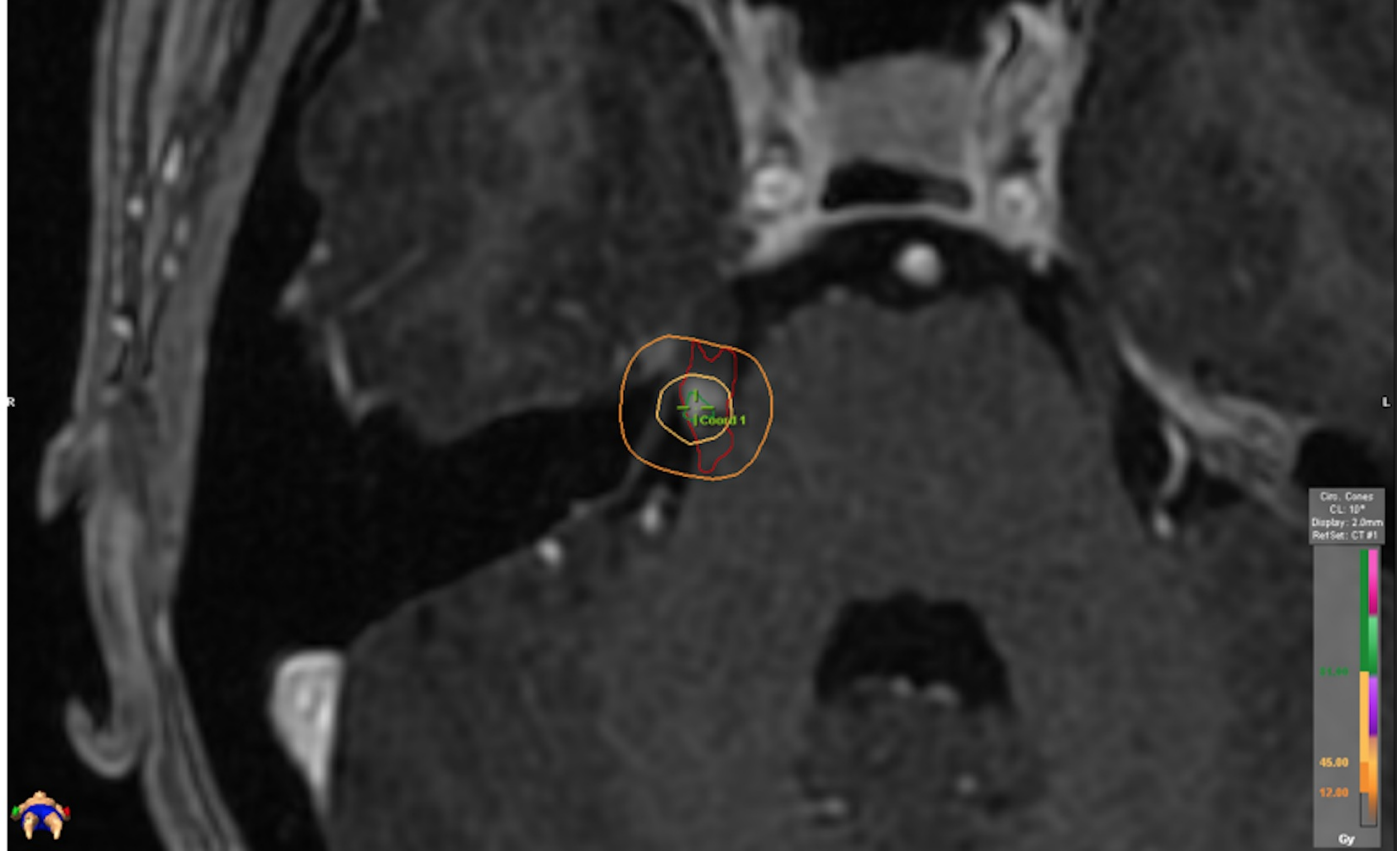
Isodose distribution with MRI changes The treatment isodose distribution of a patient with MRI changes at the root entry zone of the trigeminal nerve, depicted on a volumetric T1 image series with gadolinium contrast; note that the MRI changes remain within the 50% isodose line (45 Gy).
